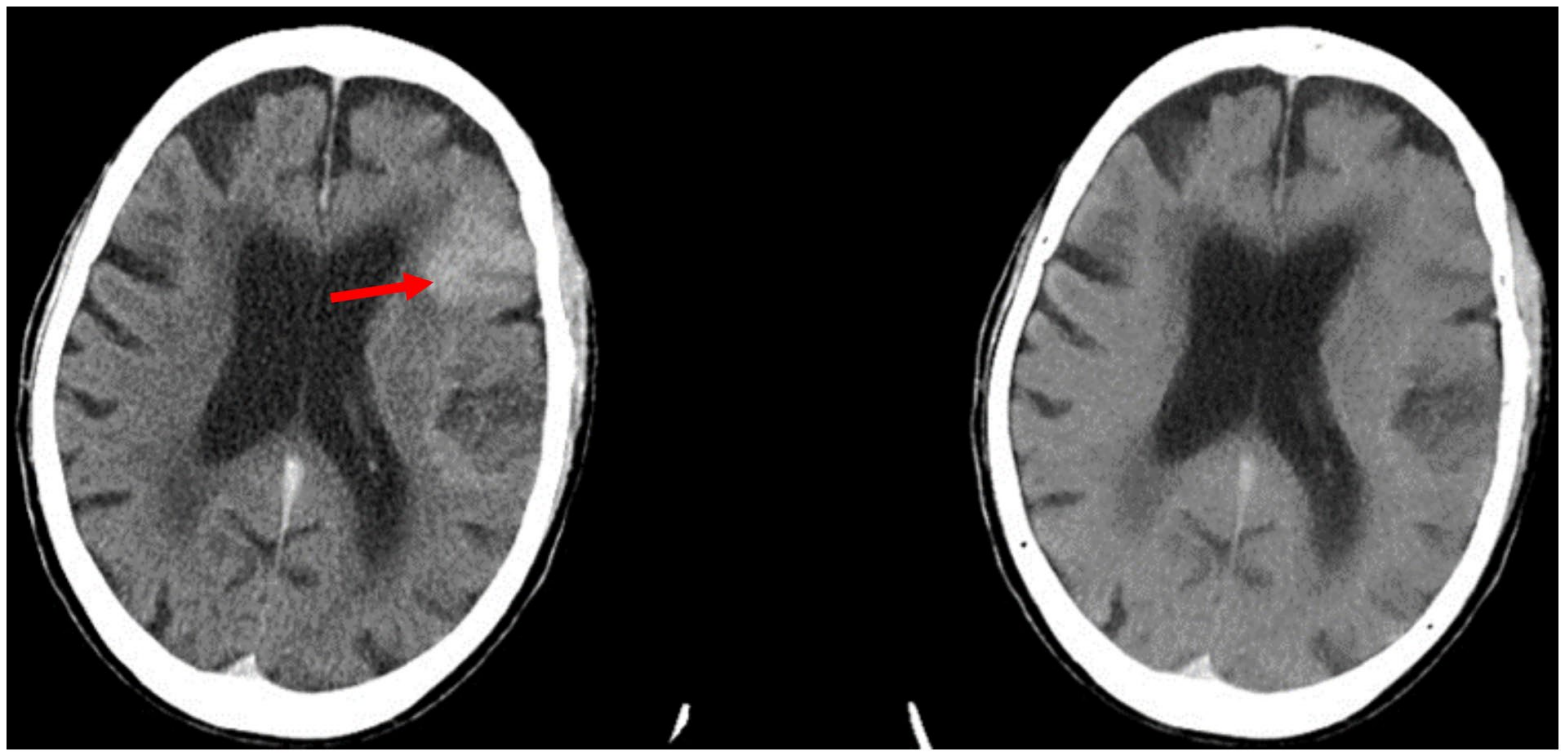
Example of a false positive reported by the Aidoc algorithm: the retained contrast in the left frontal lobe (red arrow) seen on contrast CT (left) was flagged for ICH. The iodine suppression image (right) confirms that there is no hemorrhage.
